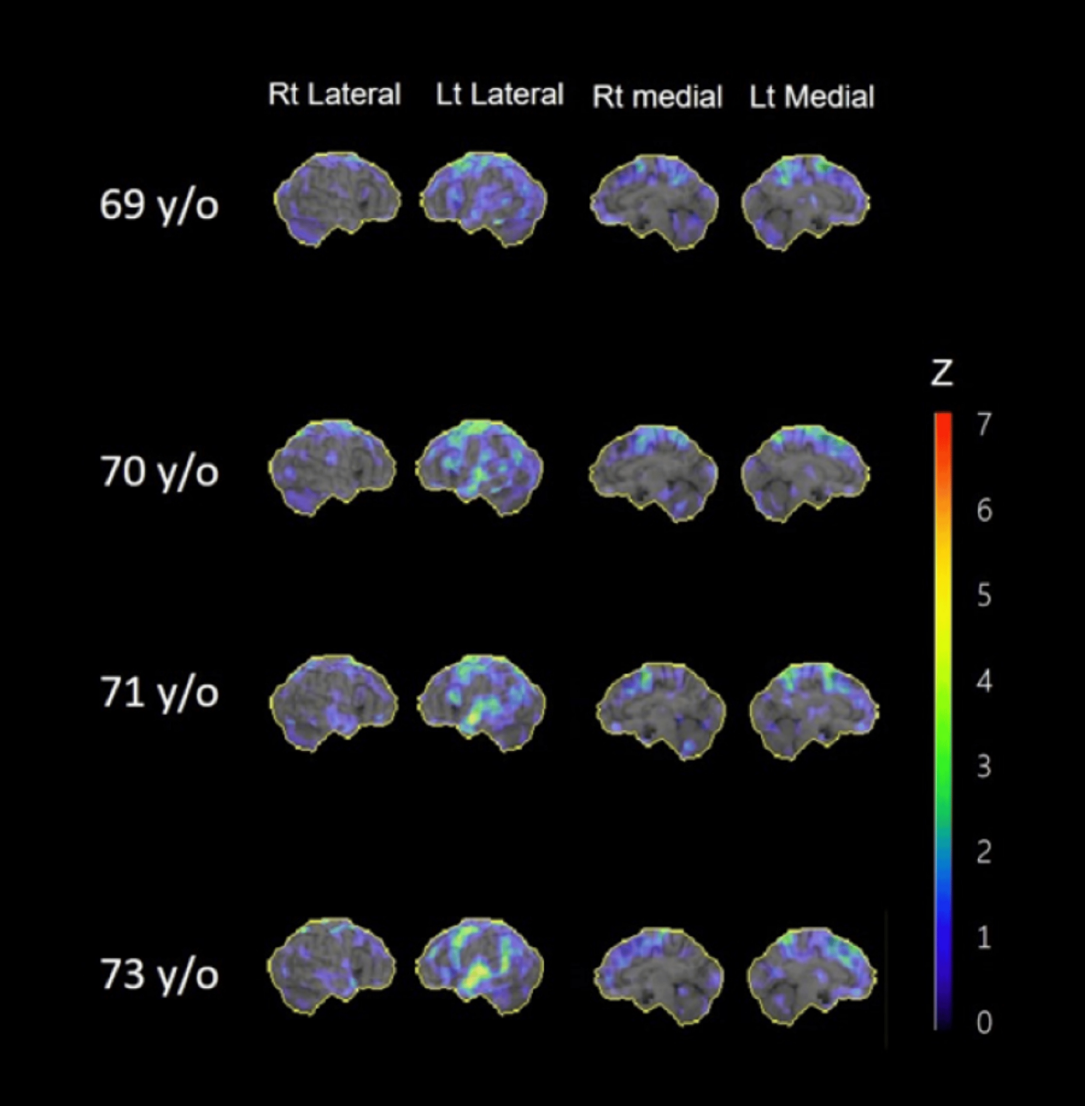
Serial results of single-photon emission computed tomography (three-dimensional stereotactic surface projection decrease). Lt, left; Rt, right; y/o, years old
